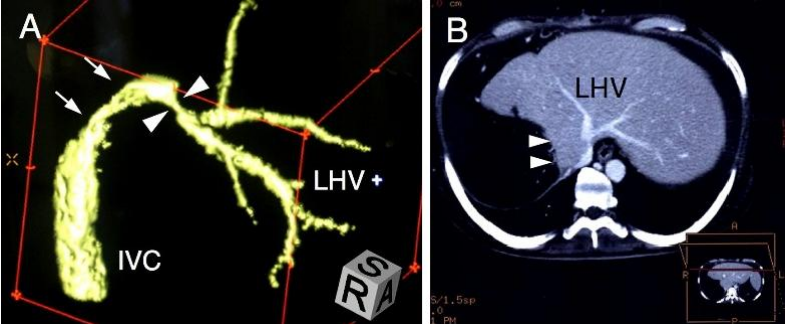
Images from computed tomography (CT) data delineating the stenosis of hepatic vein.
